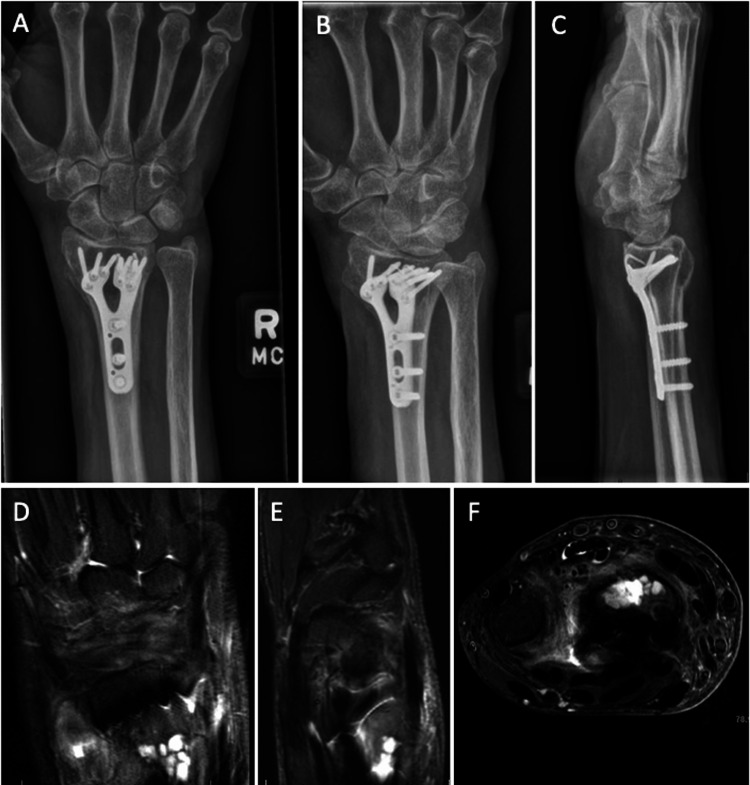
Follow-up X-rays and MRI A-C) Four-month postoperative anteroposterior, oblique, and lateral X-ray views of the right wrist: metaphyseal lucency, intact hardware, and some loss of radial height. D-F) coronal, sagittal, and axial T2 MRI sequence showing dorsal metaphyseal hyperintense lesion.
